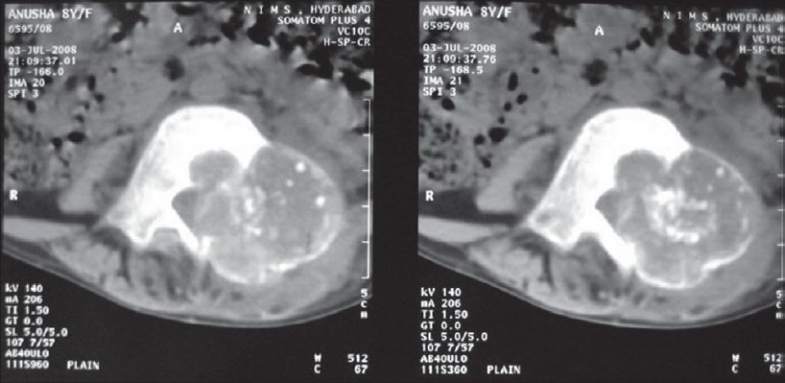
Computed tomography scan images shows expansive osteolytic lesion with thin rim of cortex involving the spinous process, lamina, pedicle, transverse process and even into the body. Calcification is well defined
